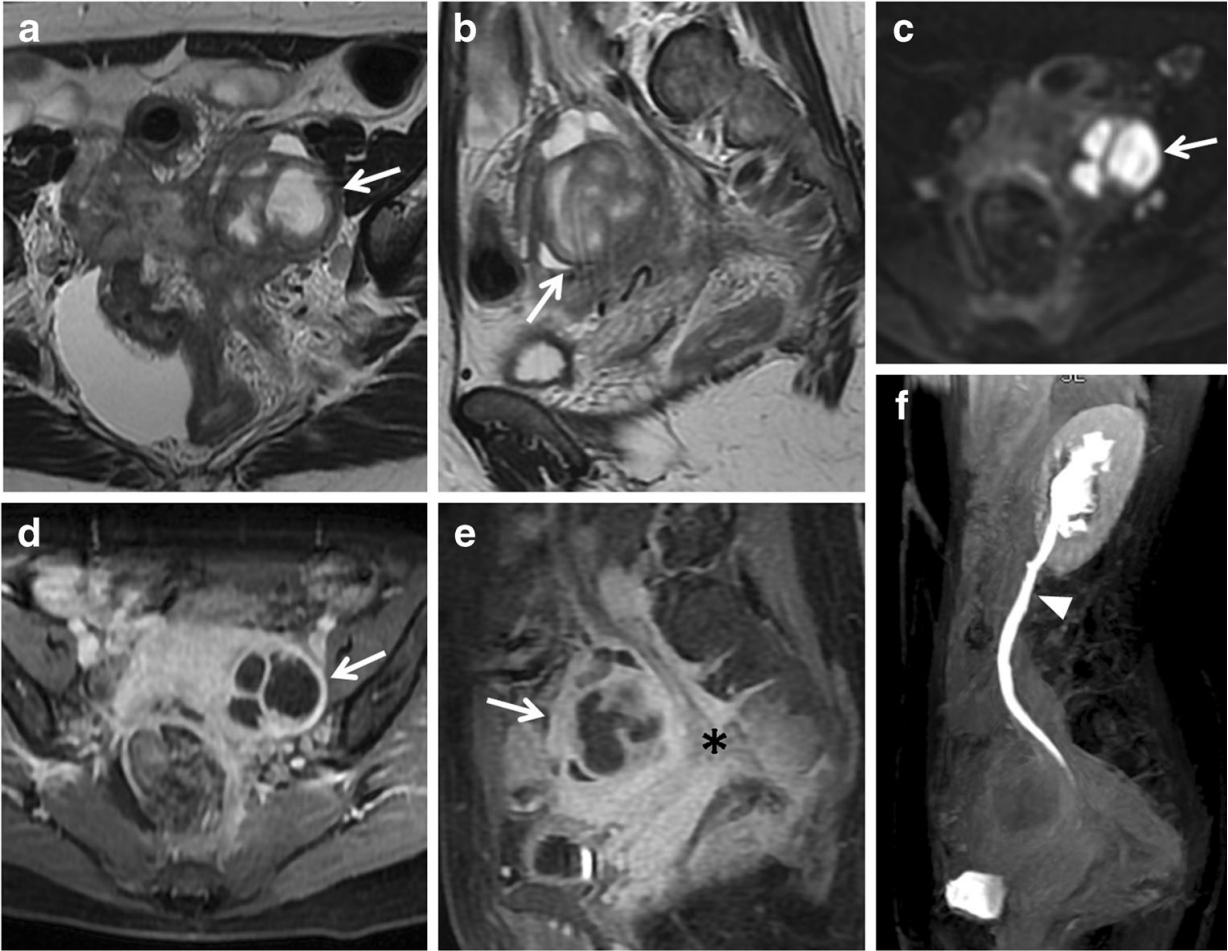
Tubo-ovarian abscess in a 53-year-old woman with acute abdominal pain and elevation of inflammatory markers such as VES and leucocytes. She has used intra-uterine devices (IUDs). (a) Axial and (b) sagittal T2-weighted images show a multilocular, cystic, pelvic mass with heterogeneous high signal intensity content on T2-weighted images (*white arrows*) in the left side of the pelvis. (c) Axial DW image (b = 800 s/mm^2^) demonstrates increased signal of the lesion (*white arrow*), a finding consistent with purulent content. (d) Axial and (e) sagittal contrast-enhanced fat-suppressed T1-weighted images show septal and thick rim enhancement in the mass (*white arrows*) and in the surrounding inflammatory stranding (*), a finding consistent with tubo-ovarian abscess. (f) Oblique sagittal MIP image (acquired about 15 min after contrast administration) shows mild dilatation of the renal pelvis and ureter (*white arrowhead*) due to the compression of tubo-ovarian abscess on the lower third of ureter
